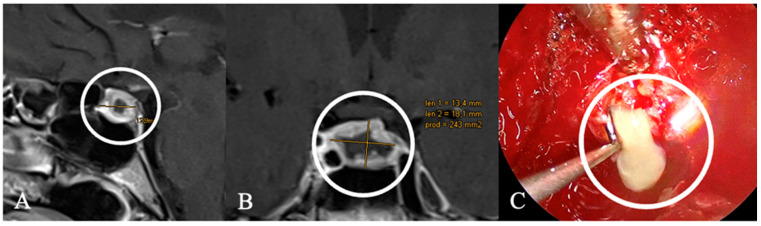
Pituitary abscess of a 61-year-old female patient. (A,B) Sagittal and coronal contrast-enhanced MRI show a contrast-enhancing intrasellar mass, with the center being necrotic. Three transsphenoidal surgeries (TSSs) for pus drainage ((C), intraoperative impression) were required, as antibiotic therapy alone was insufficient. Despite initial conservative management of meningitis, the abscess recurred, necessitating a second surgery. Over a year later, a third procedure, including partial hypophysectomy, was performed due to worsening visual disturbances and progressive hemianopsia. Cultures remained negative, and the patient received long-term meropenem treatment. After surgery and appropriate management of hypopituitarism, symptoms resolved, with significant improvement observed at the 24-month follow-up.
